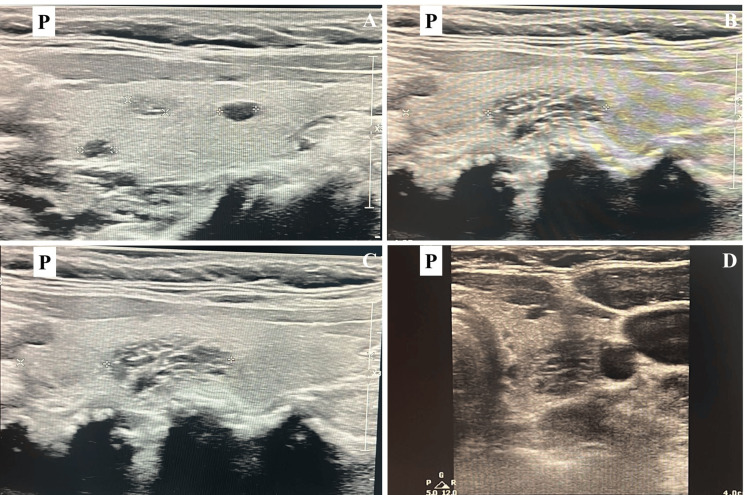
Repeated thyroid ultrasound showing stable nodules in both lobes. The consistent size and echogenicity of the nodules over three years indicate benign and nonprogressive thyroid disease (A) Right thyroid lobe: three stable hypoechoic nodules measuring 4 mm, 5 mm, and 6 mm with regular contours. (B, C, D) Left thyroid lobe: stable mixed-echogenicity oval nodule measuring 16 mm with regular margins. The “+” signs mark the boundaries of each nodule for measurement
